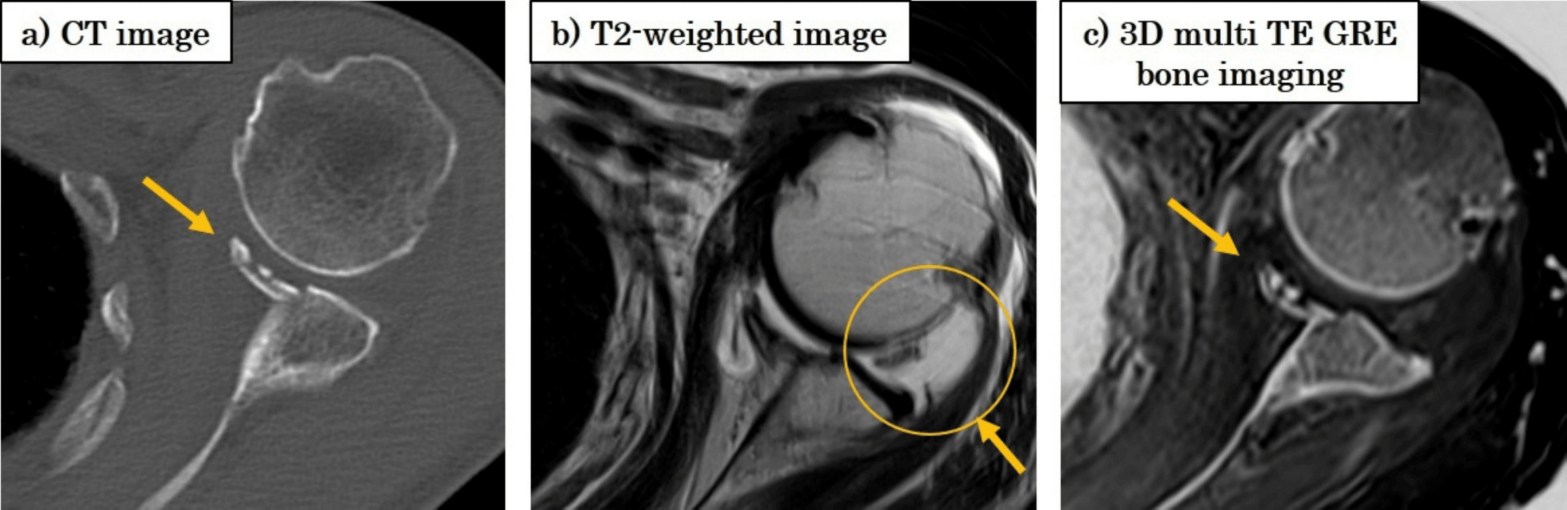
Clinical case: anteroinferior glenoid bone defect (a) CT shows the bone defect; (b) MRI shows joint effusion; (c) CT-like MRI provides a clearer depiction of the defect.
